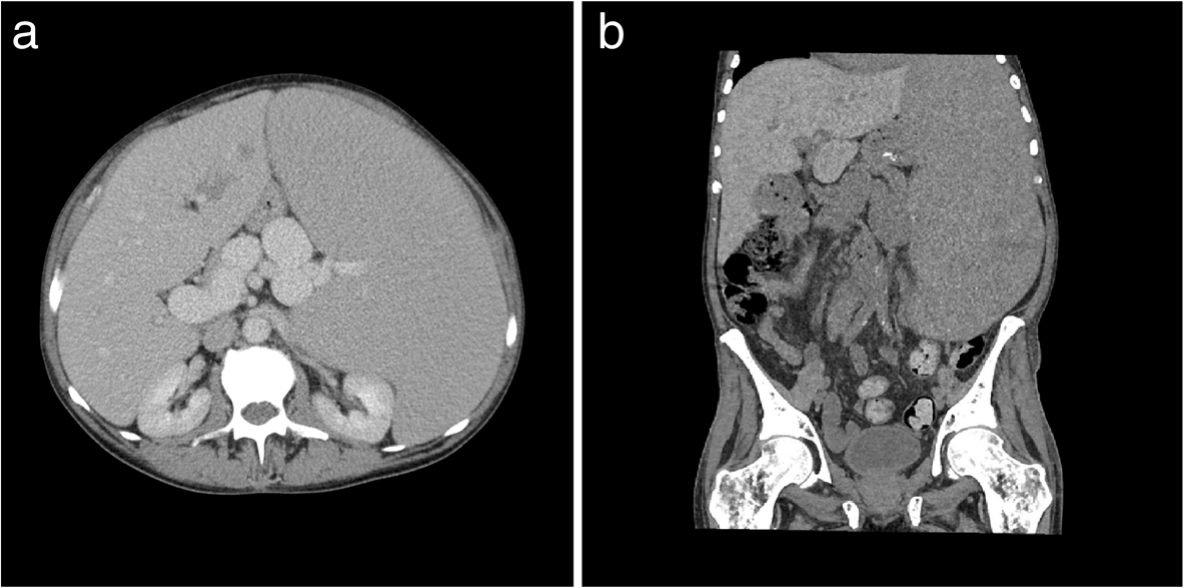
Portovenous phase axial (a) and coronal (b) CT of the abdomen in two different patients with myelofibrosis demonstrating osteosclerosis and massive splenomegaly. Note the presence of splenic varices and cavernous transformation of the portal vein on the axial image (a). Periportal low attenuation is also seen, consistent with periportal oedema and in keeping with portal hypertension. On the coronal image, linear low attenuation at the inferior aspect of the spleen is consistent with splenic infarction, another complication of massive splenomegaly
